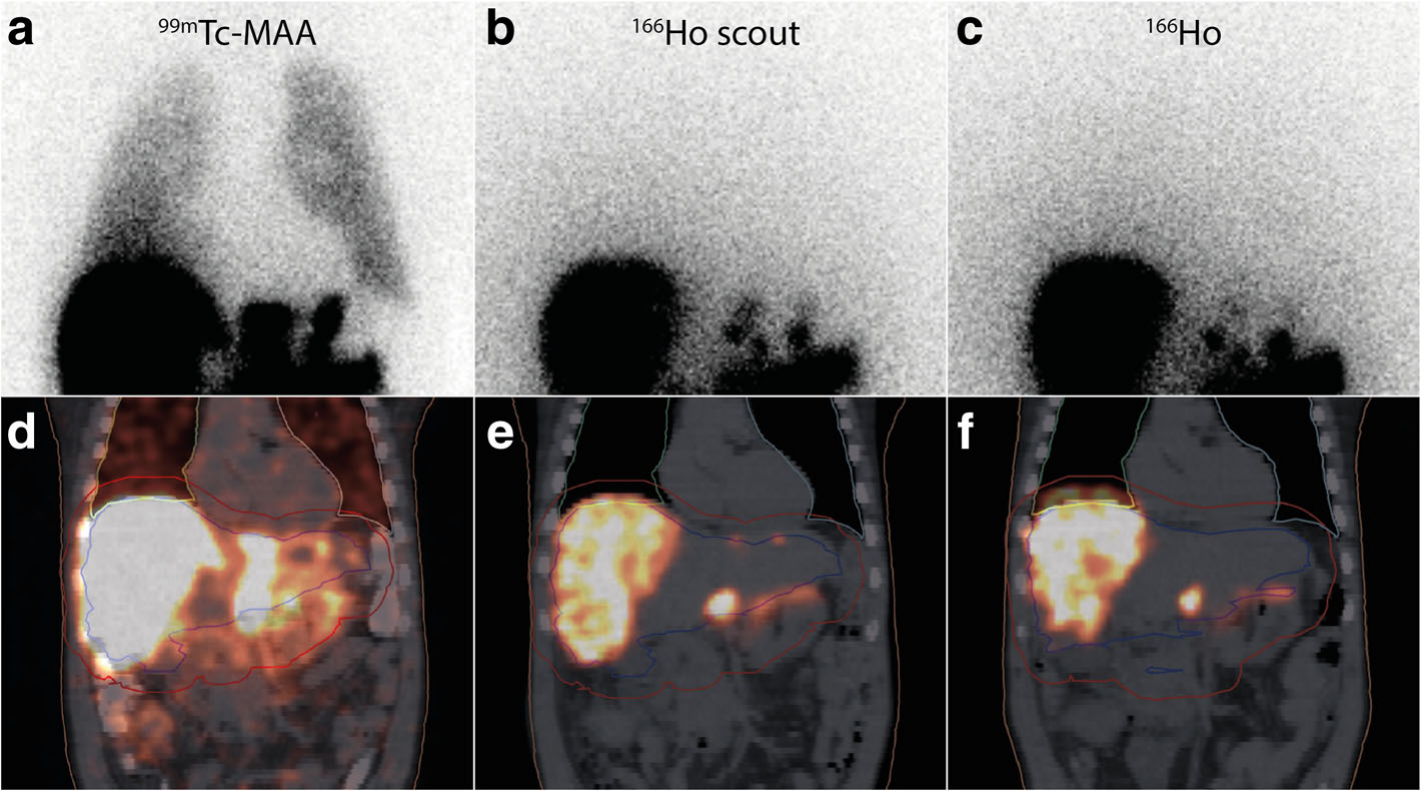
Example of lung shunt fraction overestimation by ^99m^Tc-MAA. A patient with multiple liver metastases of a cholangiocarcinoma treated in the prior HEPAR 2 trial. Note the (visual) significant overestimation of ^99m^Tc-MAA on planar imaging compared to the ^166^Ho-scout dose and ^166^Ho-treatment dose. Quantification of the lung shunt fraction on planar and SPECT/CT imaging confirmed the visual assessment: a
